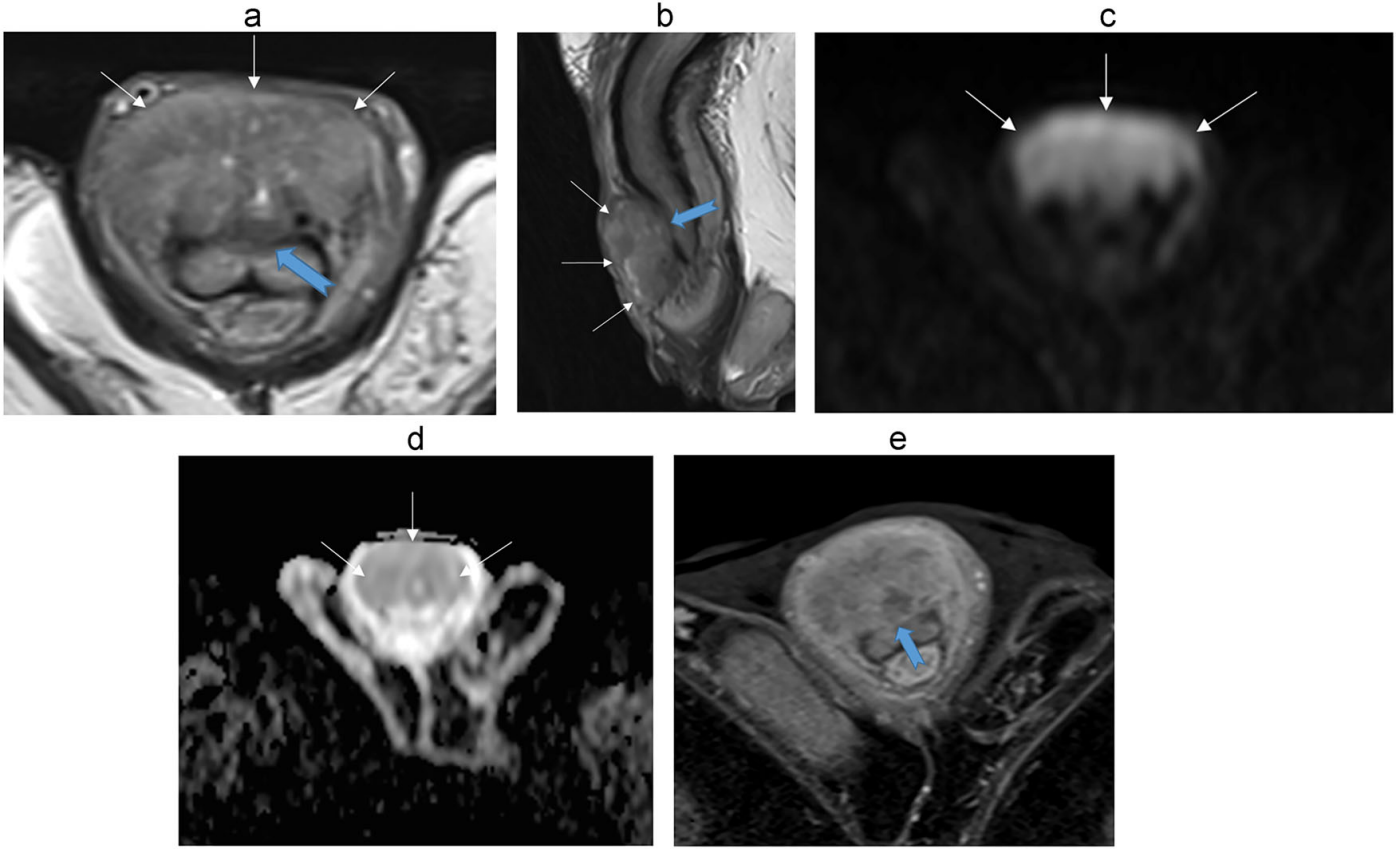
Seventy-five-year-old patient was referred for a large lesion of the glans. a Axial, (b) sagittal T2-W MR images show a tissue lesion (three white arrows) with intermediate signal. c Axial DWI and (d) ADC map images show intense diffusion restriction. Disruption of the inner layer of the T2 dark line of albuginea (blue arrow) on axial T2 sequences and on (e) enhanced T1 sequence in favor of a cavernosal invasion. Stage pT3N0
